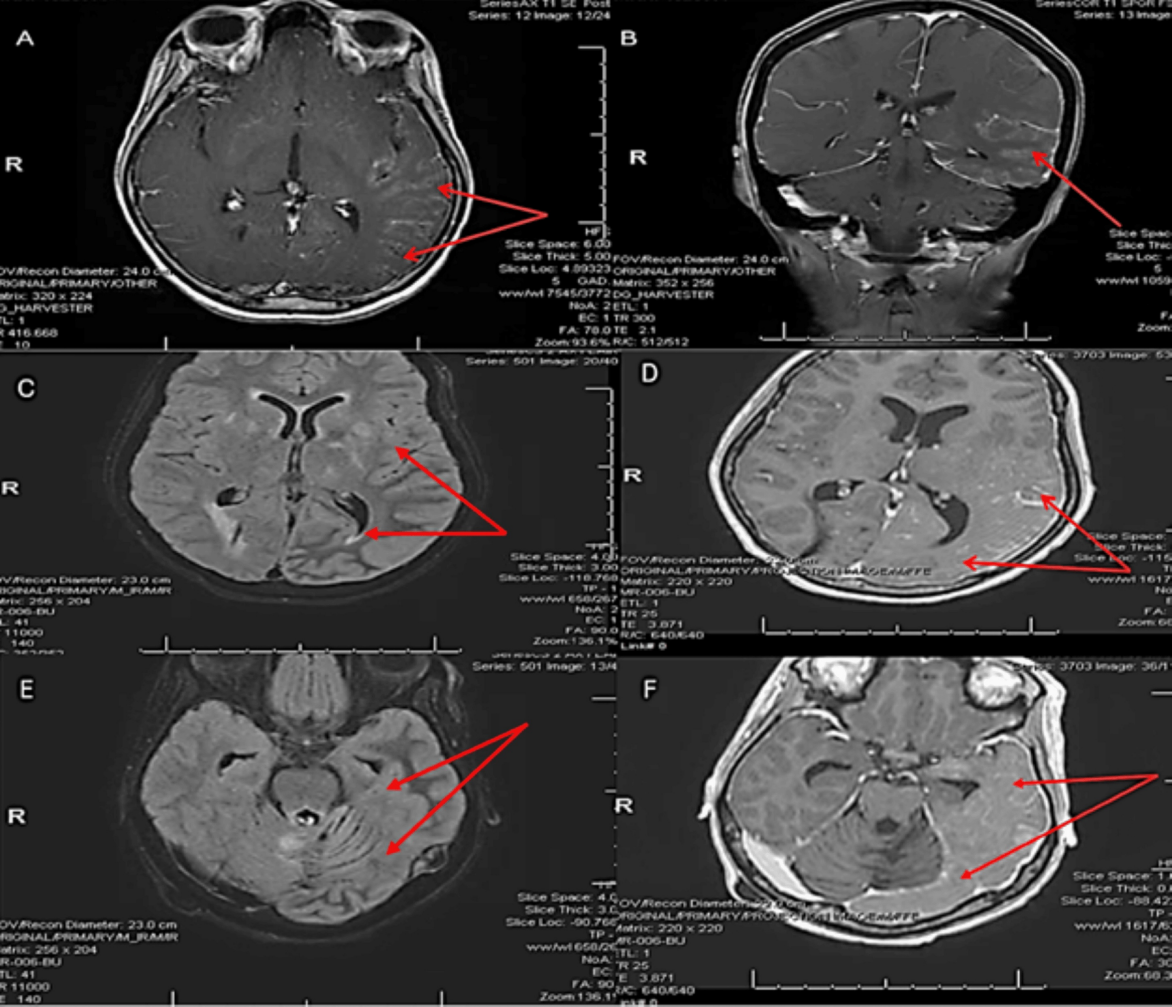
Axial T1-weighted SE sequence with contrast showing diffuse left temporoparietal and occipital leptomeningeal and cortical enhancement in the region of prior radiation therapy (red arrows) (A) and corresponding enhancement of the left temporal lobe as seen on coronal view (B). T1 FLAIR without contrast in the aforementioned regions (C and E) and their corresponding findings on T1-weighted sequence with contrast.
